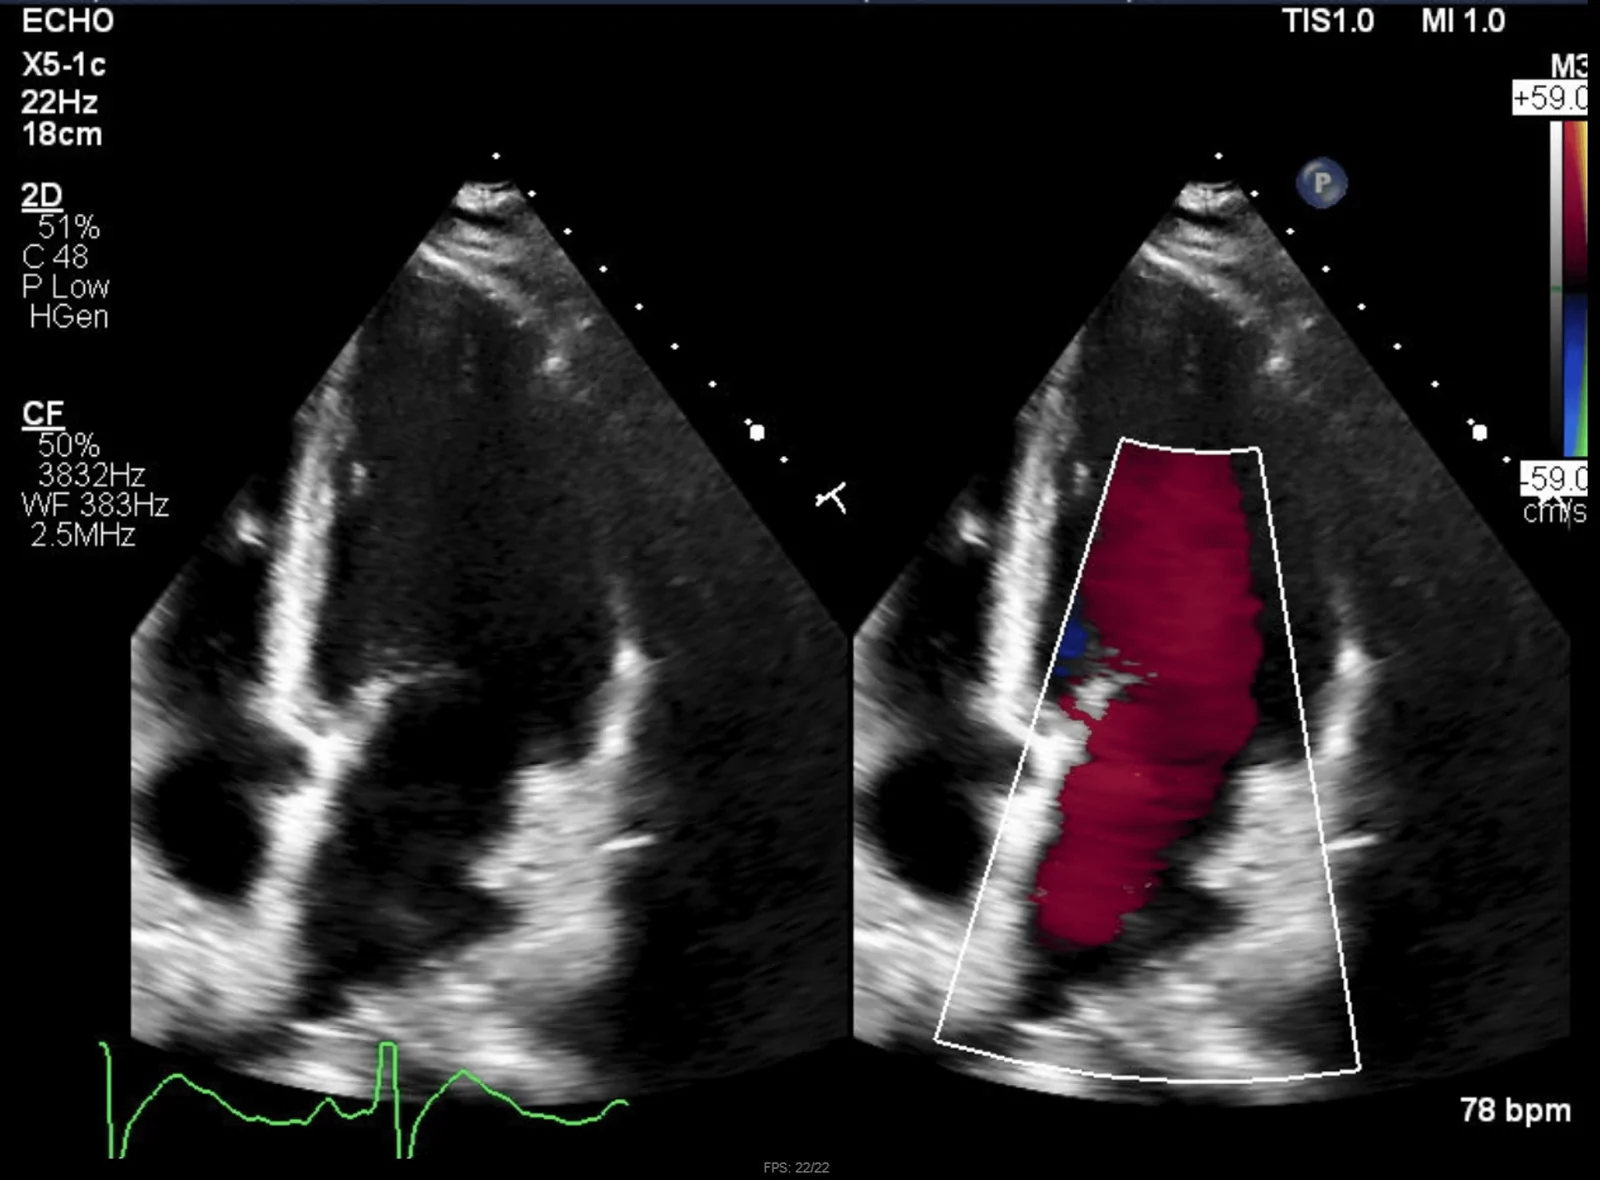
Transthoracic echocardiogram obtained after hemodynamic deterioration, demonstrating a moderately dilated left ventricle with severely reduced systolic function (estimated ejection fraction 25%-35%).
